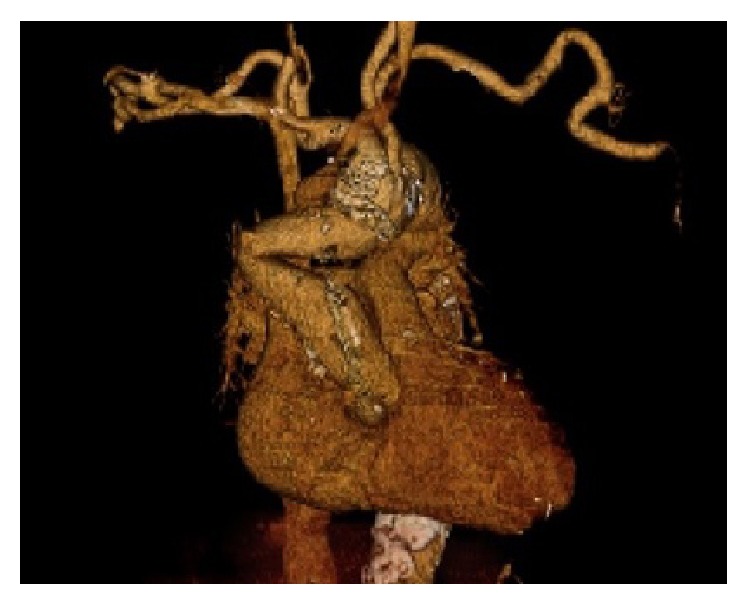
Chest 3-dimensional computed tomography. Computed tomography revealed that the replaced duct was severely bent in the ascending aorta.
